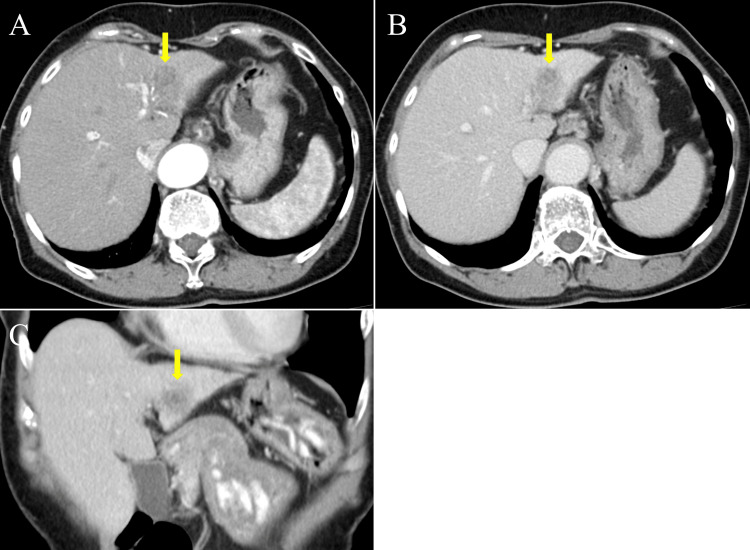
CT images seven months after HCC diagnosis A-C: Follow-up CT images showed marked regression of the previously hypervascular hepatocellular carcinoma (HCC) with washout, which had occupied the entire left lobe. Only a residual low-attenuation area, suggesting a possible remaining lesion, was observed in the lateral segment of the left lobe (shown by the yellow arrow). The tumor thrombus in the left branch of the main portal vein had disappeared.
